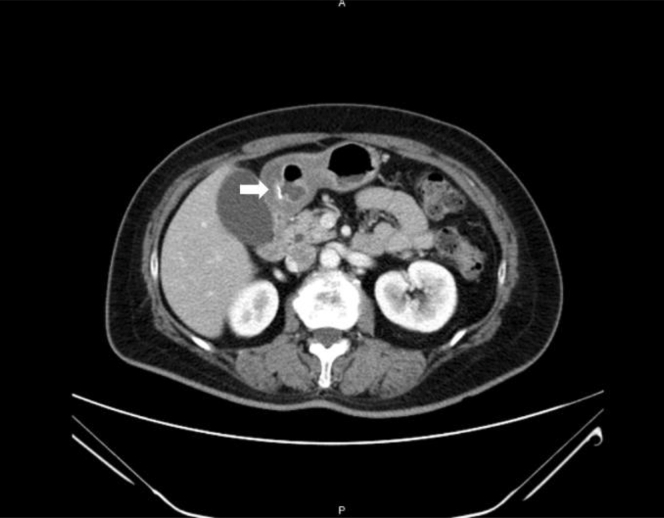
Computed tomography image of the abdomen reveals a hyperdense linear foreign body (arrowhead) in the gastric antrum.
